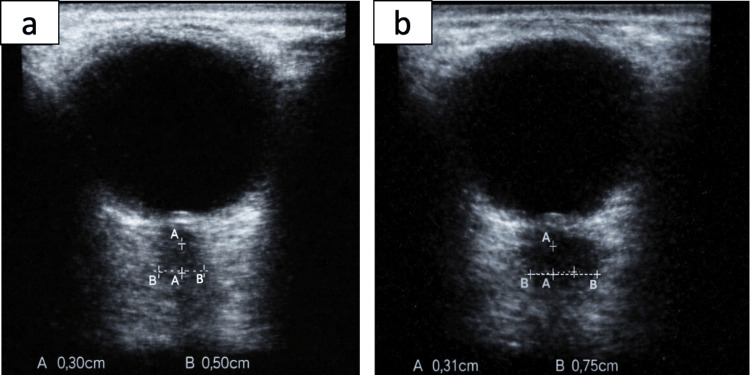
Ultrasound optic nerve sheath diameter measurement (diameter represented as B caliper length): (a) right eye and (b) left eye
